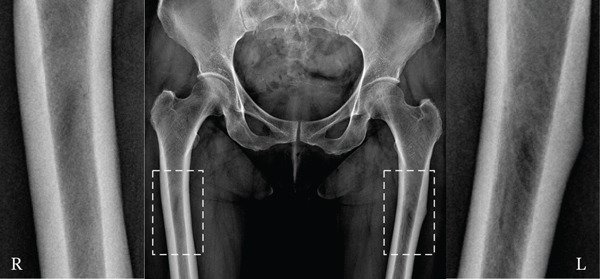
Anteroposterior radiographs of the bilateral hip joints and femurs at the initial consultation.
